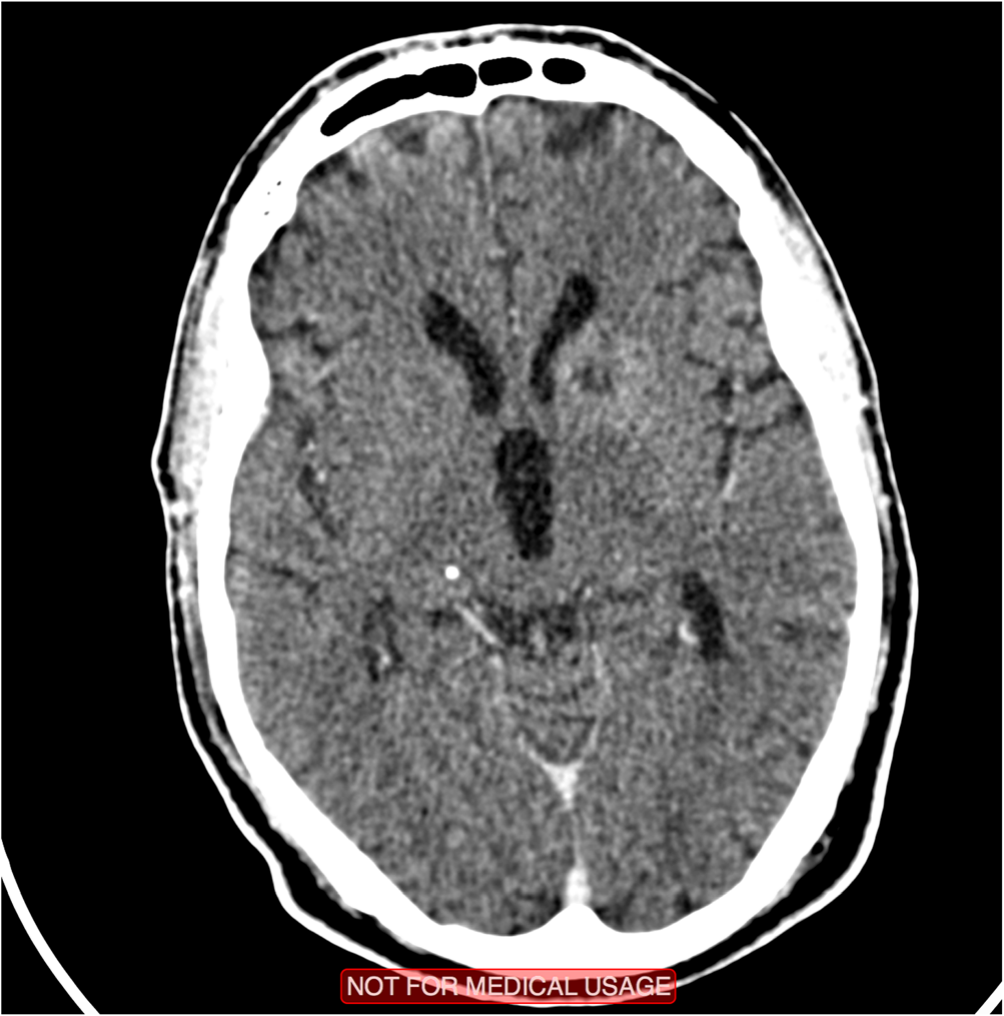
Computerised tomography (CT) of the head with evidence of probable perforator territory infarction on the left in an HIV-1-infected patient with tuberculous meningitis.
